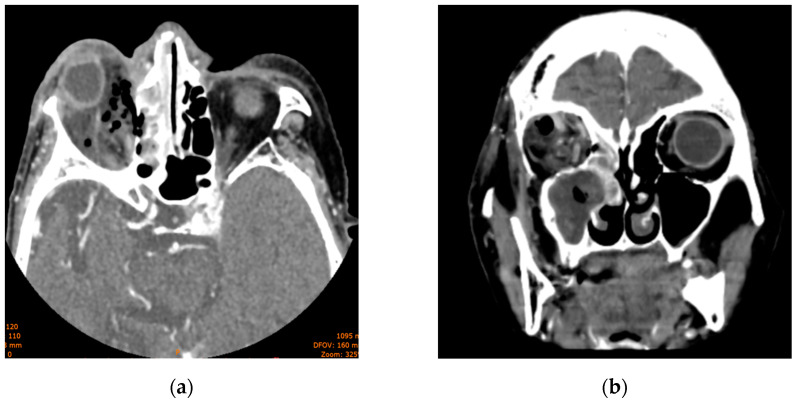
Computed tomography scan displaying maxillary and ethmoid sinus opacification, multiple gas bubbles extending from the ethmoidal cells to the right orbit, a stretched right optic nerve, and posterior globe tenting: (a) axial section; (b) coronal section.
